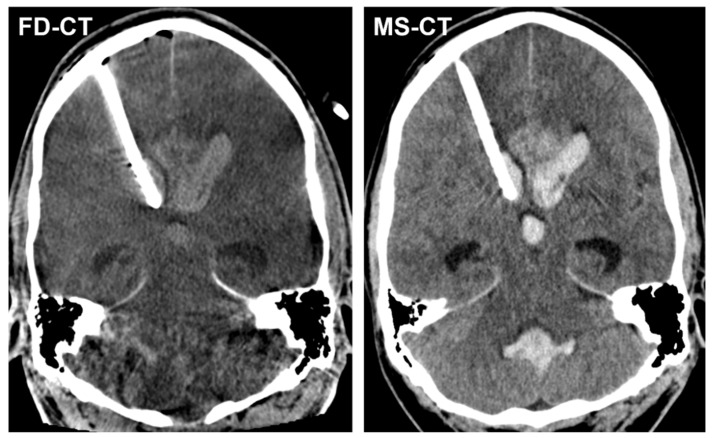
Exemplary FD-CT and MS-CT images of a patient with an endovascular treated, aneurysmal SAH and implanted external ventricular drain (EVD) because of acute obstructive hydrocephalus (AOH). Both FD-CT and MS-CT allow the precise determination of the intraventricular EVD position in the frontal horn of the right lateral ventricle as well as the delineation of the dilated ventricular system.
